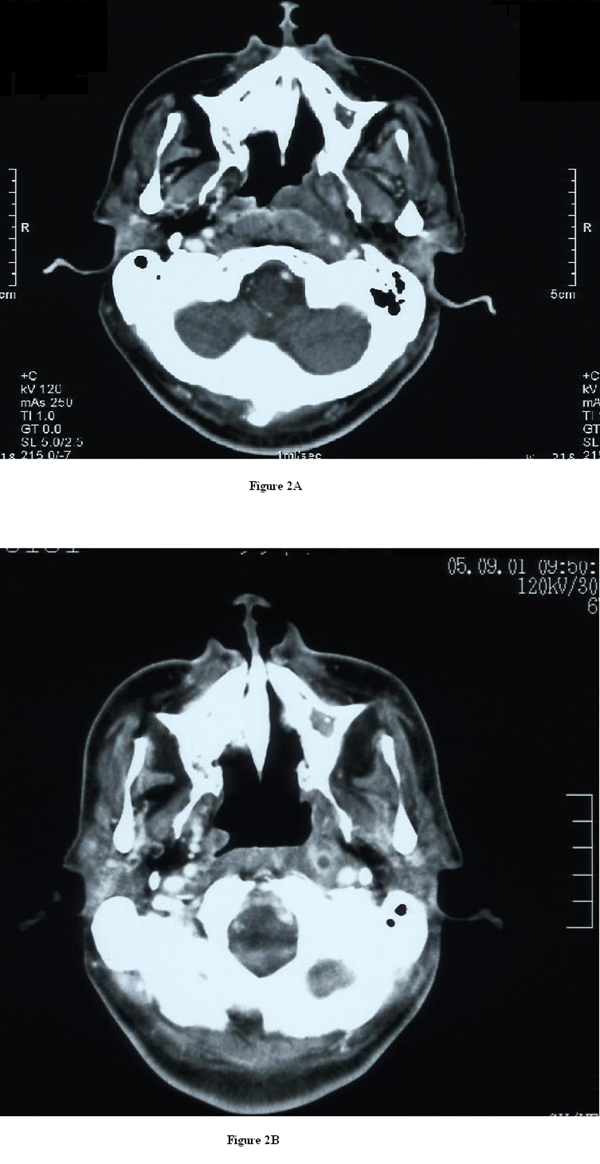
(A) Computed tomography of the neck with intravenous contrast showing the left cellulitis at the level of the nasopharynx (white arrow). (B) Computed tomography examination (6 weeks after surgery) indicated the presence of a necrotic retropharyngeal lymph node at the level of the nasopharynx (white arrow).
